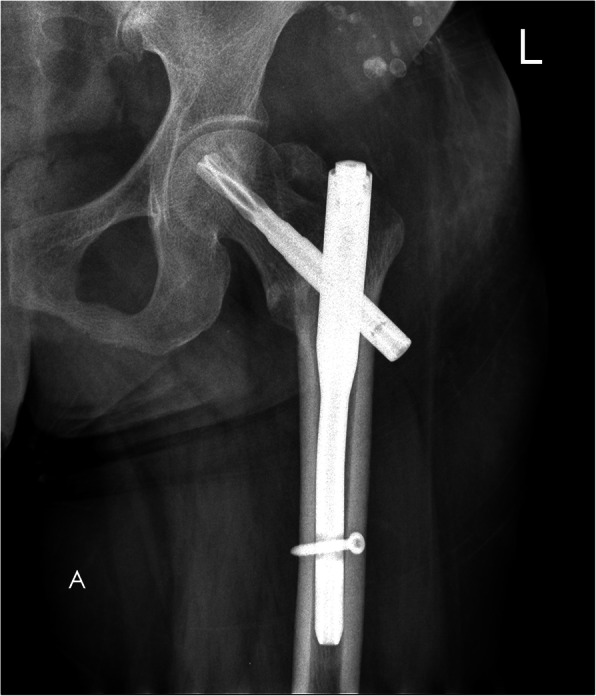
The anteroposterior radiograph of left hip after the PFNA fixation
